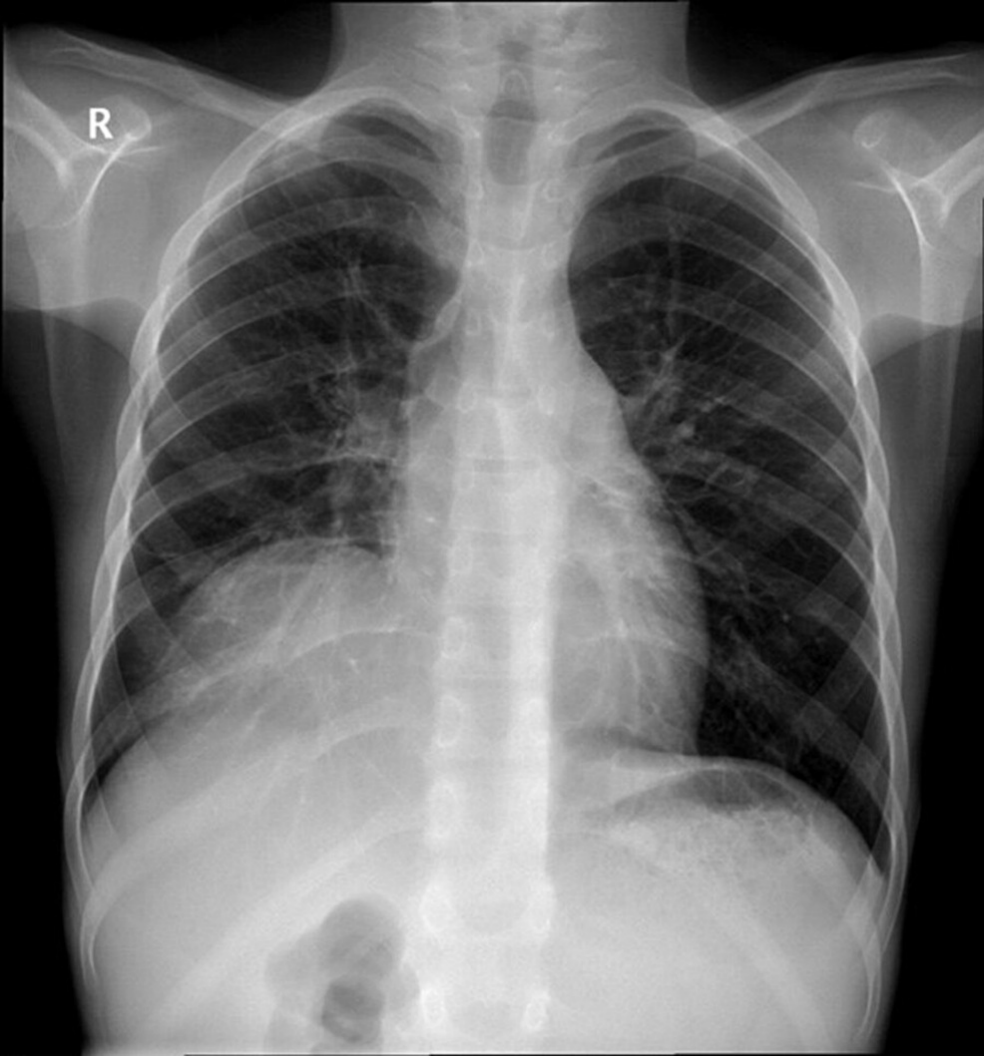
Chest x-ray, AP view, showing opacity in the right lower lung with vascular markings, normal cardiothoracic shadow with no obliteration of the costophrenic angles.
